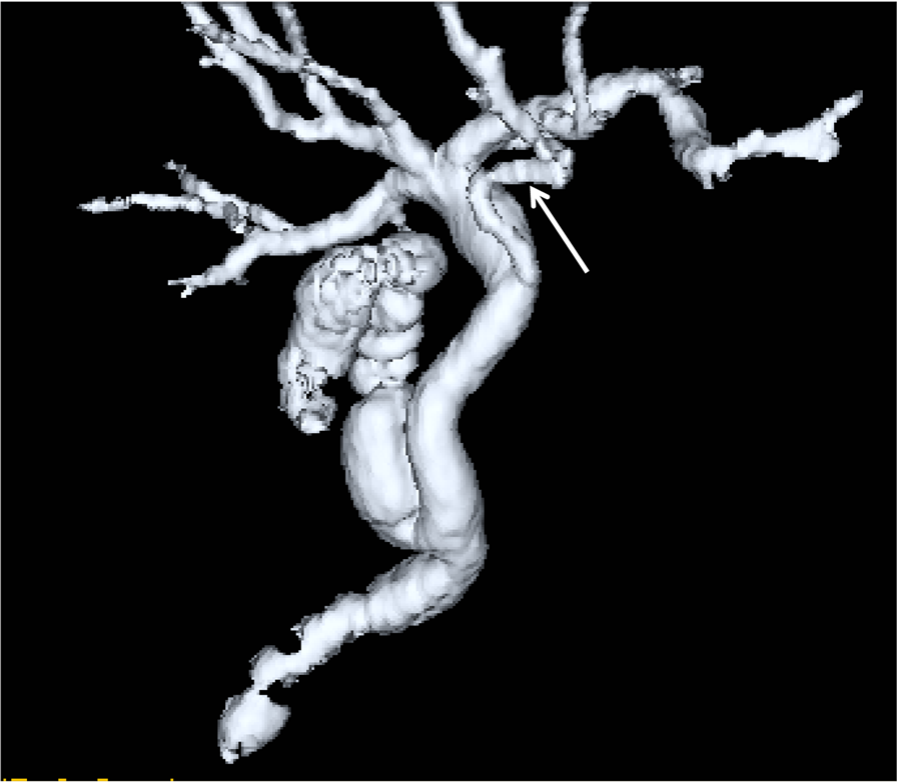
Three-dimensional DIC-CT image (ventral view) showing the type V accessory hepatic duct. The accessory hepatic duct drained segment 4 (*arrow*) and was classified into the left-sided branch type
